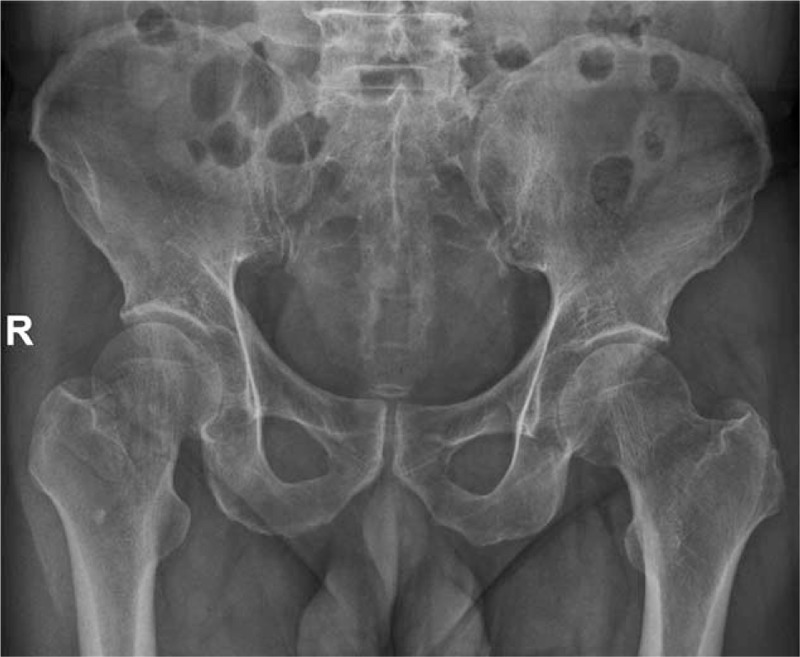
X-ray of right hip. A 42-year-old male patient suffered from a high falling injury. According to the preoperative x-ray image, the discontinuity of bone in the right femoral neck was observed, and femoral neck fracture was initially diagnosed.
